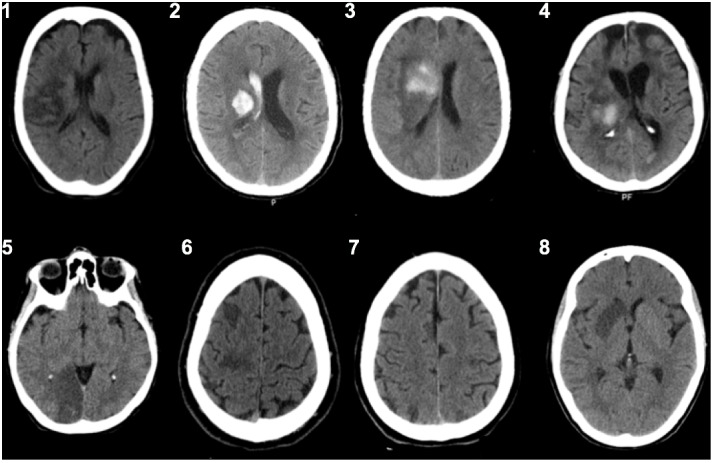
CT scans from each patient (patient ID 1–8, see Table 1) at time of assessment.
